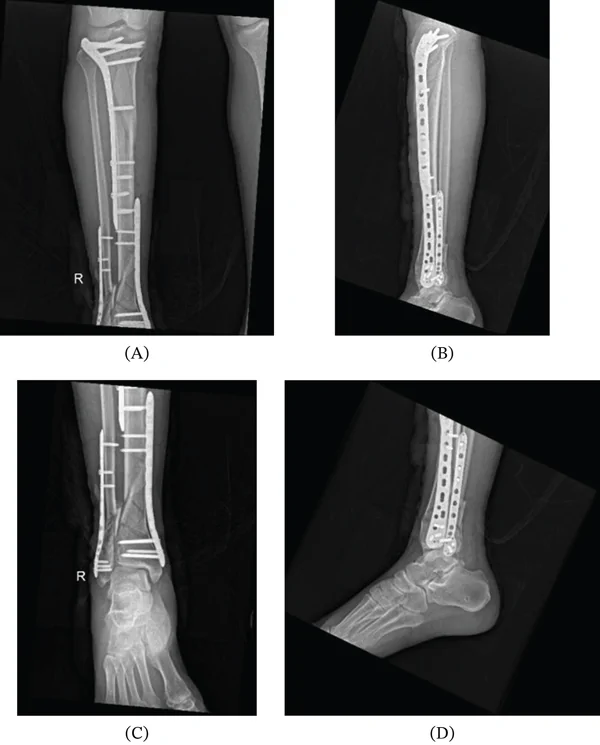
Postoperative radiographs. (A) Anteroposterior and (B) lateral views of the tibia demonstrate lateral locked‐plate fixation of the proximal tibia and plate fixation of the fibula. (C) Anteroposterior and (D) lateral ankle views demonstrate percutaneous screw fixation of the distal tibial plafond component and restoration of coronal and sagittal alignment. Immediate postoperative plain‐radiograph measurements were MPTA 87°, LDTA 89°, and posterior distal tibial angle 78°. Residual articular incongruity was estimated as < 1 mm on the available intraoperative/postoperative radiographic assessment; no postoperative CT is available for confirmation.
